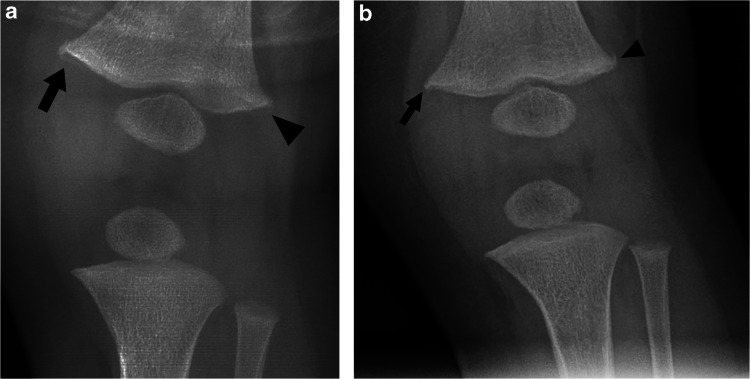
A subtle classic metaphyseal lesion (CML) in a 7 month-old boy with a challenging follow-up radiograph mimicking a metaphyseal variation. a. Anteroposterior (AP) radiograph shows a distal left femoral subtle bucket handle fracture in the medial (arrow) and lateral metaphysis (arrowhead) suggestive of CML. b. Fifteen-day follow up AP radiograph shows distal femur medial corner irregularity (arrow) and lateral metaphysis spur (arrowhead). Six radiologists independently reviewed the initial and follow-up radiographs with moderate to high confidence, blinded to clinical history. The three pediatric radiologists diagnosed a CML initially and a metaphyseal variation on follow-up, while the three adult emergency radiologists diagnosed a metaphyseal variation initially; on follow-up, two diagnosed again metaphyseal variation and one read the study as normal
